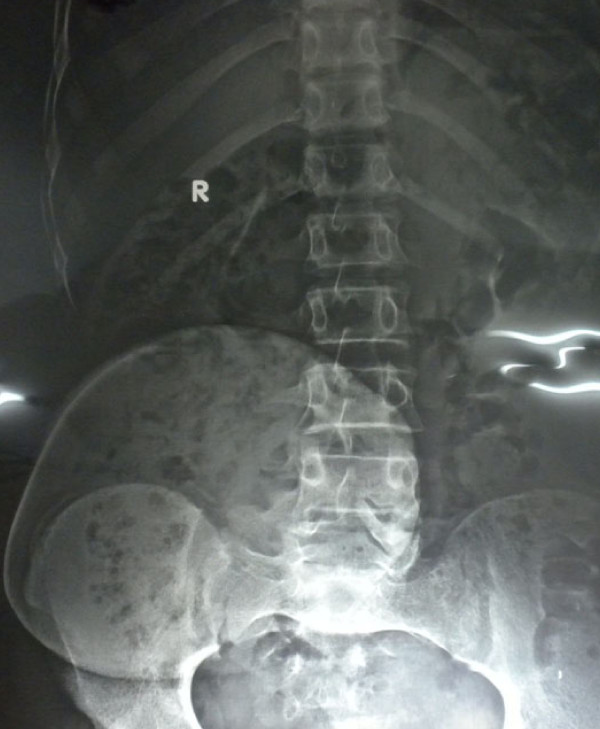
Radiograph showing the well-defined outline of the teratoma.
